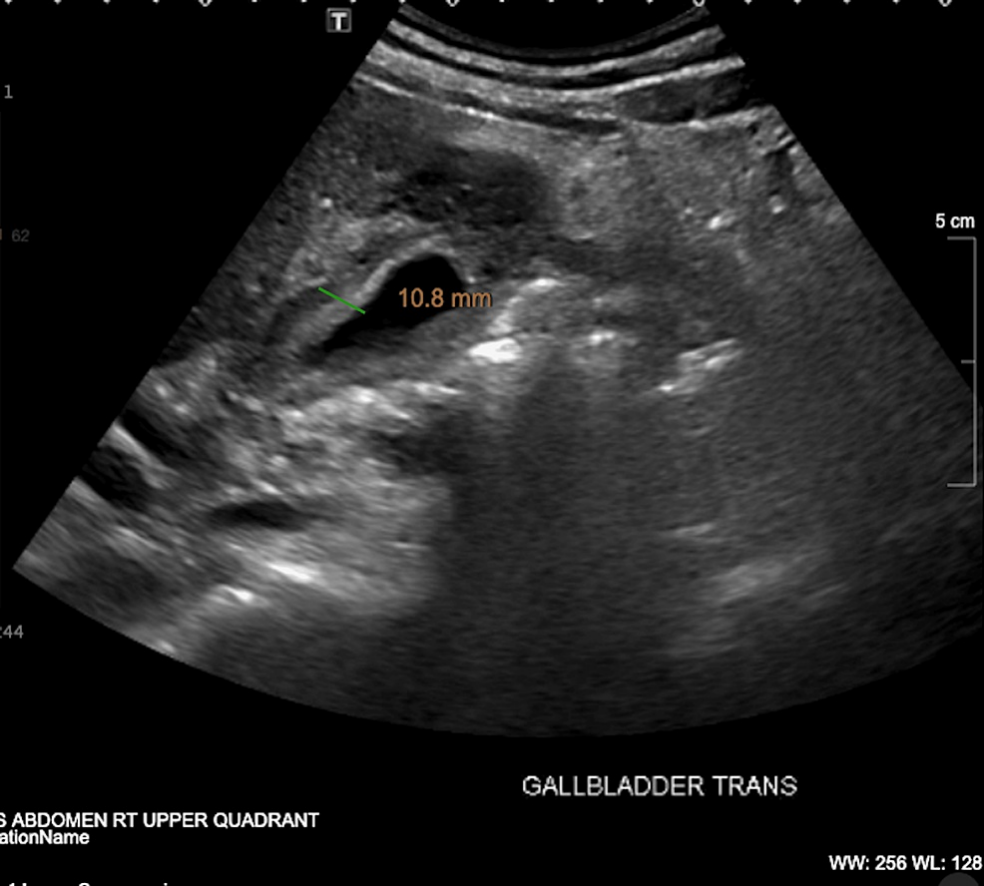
RUQ ultrasound shows diffuse gallbladder wall thickening Gallbladder wall hematoma versus cholecystitis, though less likely RUQ: right upper quadrant
